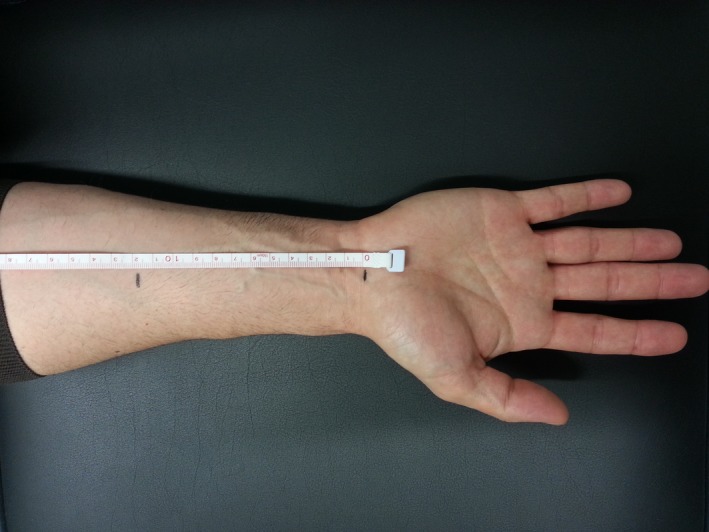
The two forearm levels where median nerve cross‐sectional area are measured by high‐resolution sonography: 12 cm proximally from wrist (R/L12) and directly above the wrist crease (R/L0).
